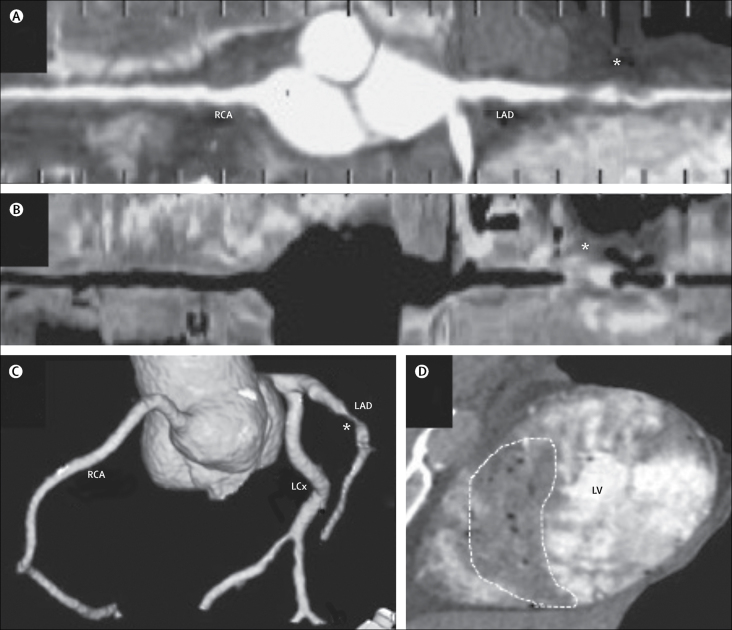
Images from post-mortem CT with targeted coronary angiography in a case of myocardial infarction Post-mortem CT with targeted coronary angiography (PMCTA) of a male ex-smoker aged 62 years with borderline type 2 diabetes who died suddenly and unexpectedly. PMCTA reconstructed images with straightened curved multiplane reconstructions of the positive (A) and air (B) contrast runs, and a 3D-volume reformat (C), all showing a critical stenosis of the proximal left anterior descending artery, as indicated by an asterisk. The myocardium showed an anteroseptal perfusion deficit, indicated by a dashed line (D). In the absence of artery calcification, these findings were diagnosed as a soft plaque occlusion leading to acute myocardial infarction. RCA=right coronary artery. LAD=left anterior descending. LCx=left circumflex. LV=left ventricle.
